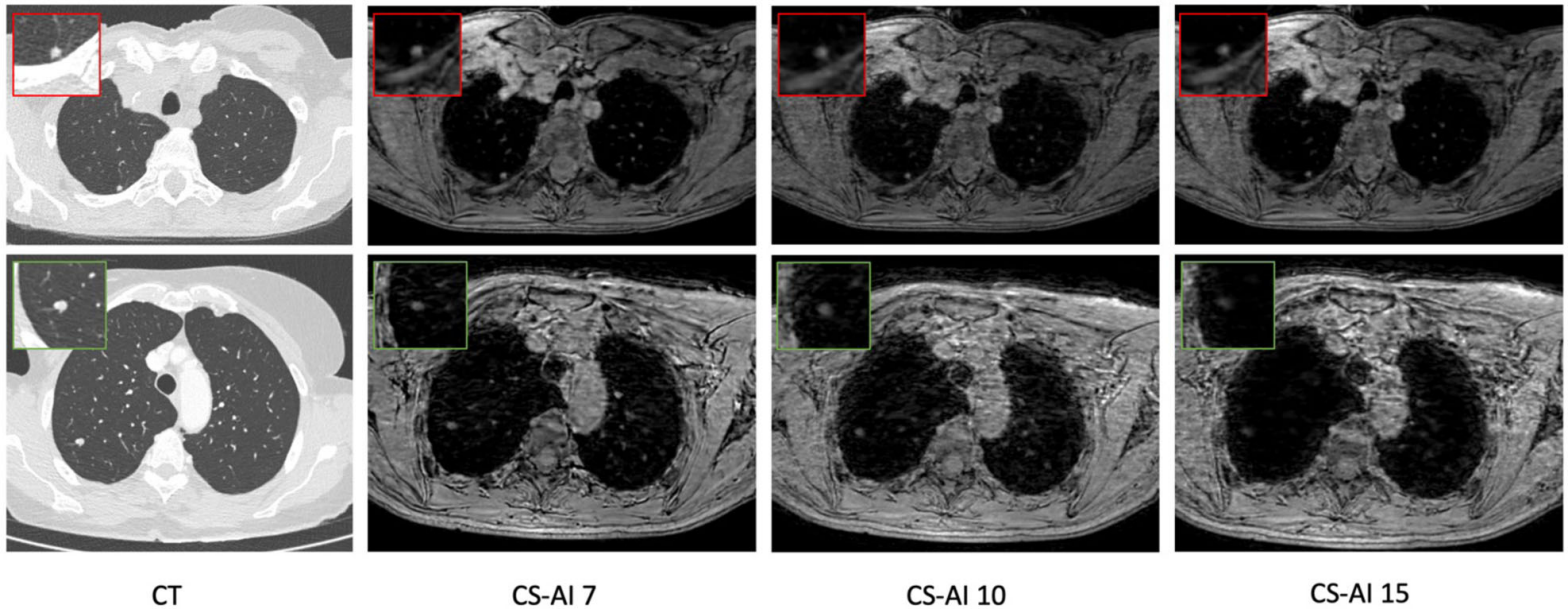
Exemplary CT images and MR images with different acceleration factors CS-AI-7, CS-AI-10, and CS-AI-15. The top row shows a case with a high score for image quality and morphology for all acceleration factors, whereas the bottom row shows a case with low image and morphology quality for CS-AI-10 (image quality 3; morphology slightly inferior) and 15 (image quality 2.5; morphology substantially inferior)
